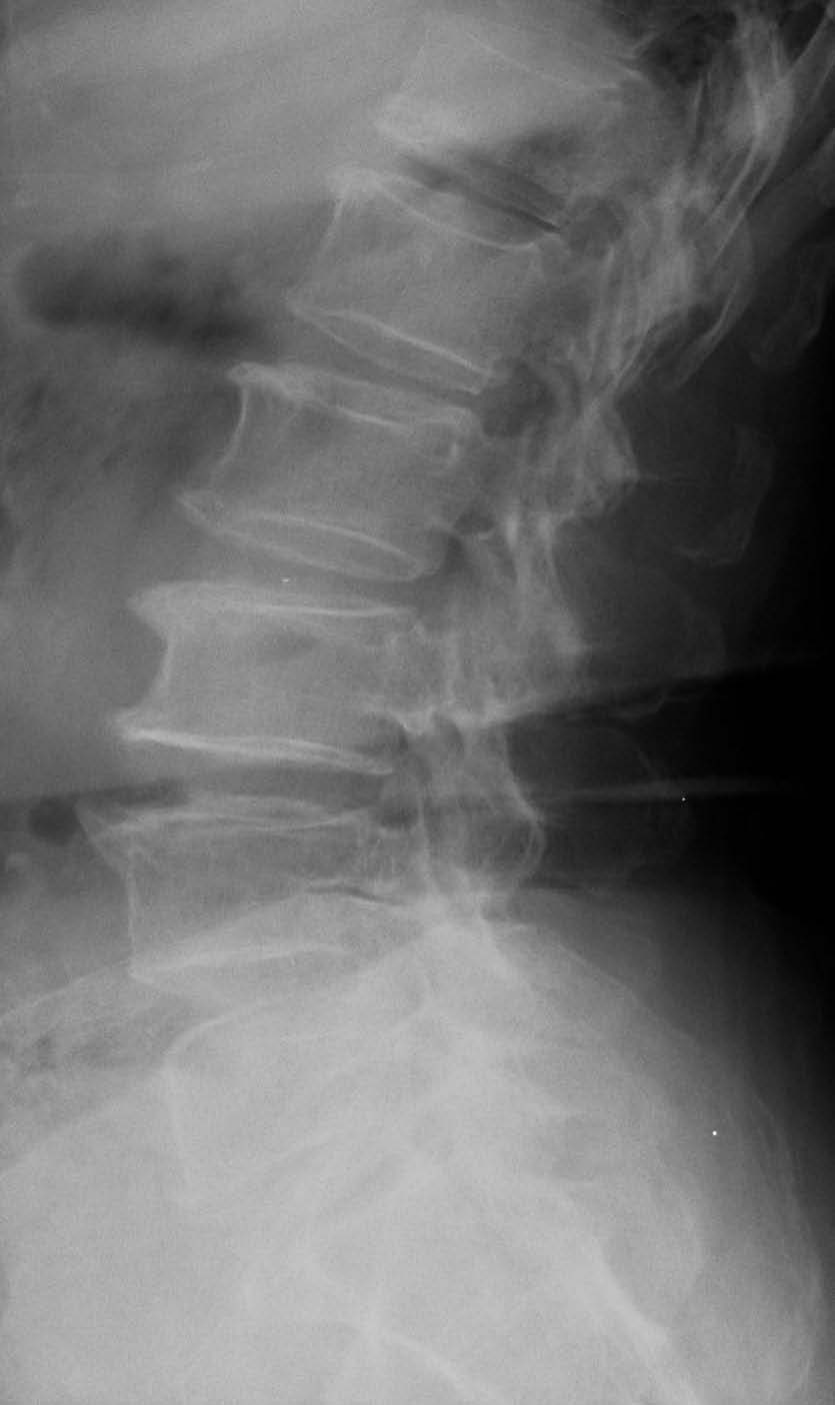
Osteophytosis and DSN demonstrated on a lateral lumbar spine X-ray.
